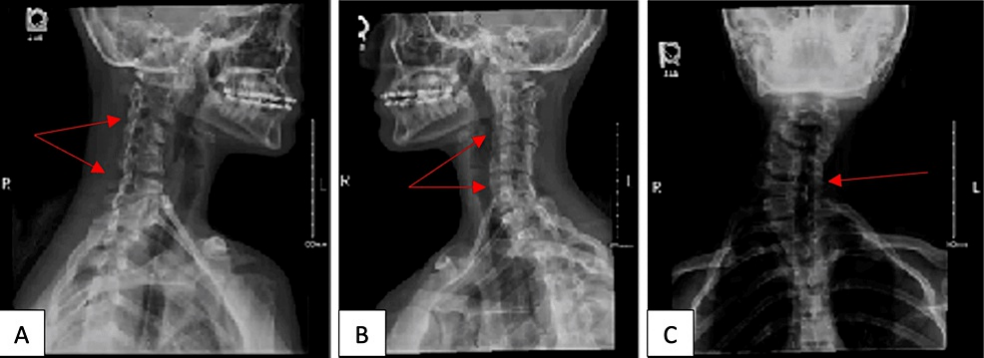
X-ray of the cervical spine (A) Partial fusion of C2-C5 and C6-C7 (arrows). (B) Partial fusion of C2-C5 and C6-C7 (arrows). (C) Scoliosis of the cervical spine and widening at C5-C6 (arrow).
